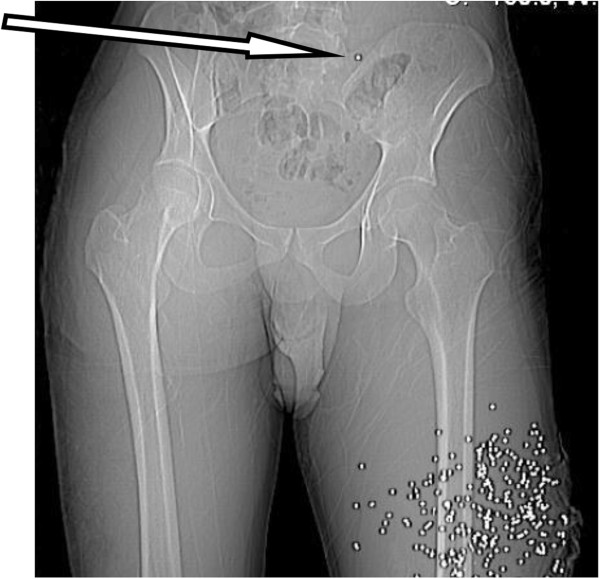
CT scout view pelvis and upper thigh.
